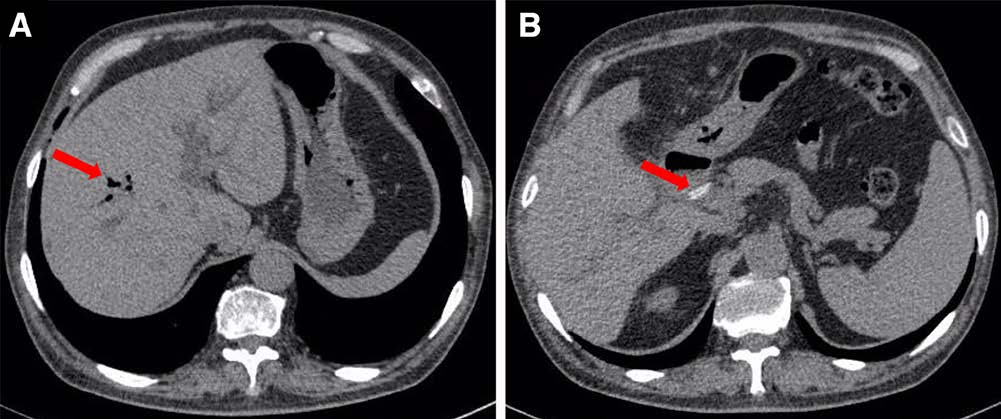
A and B are preoperative CT scans of the upper abdomen; the red arrows in A and B represent gas and hemlock clamps, respectively. CT = computed tomography.
